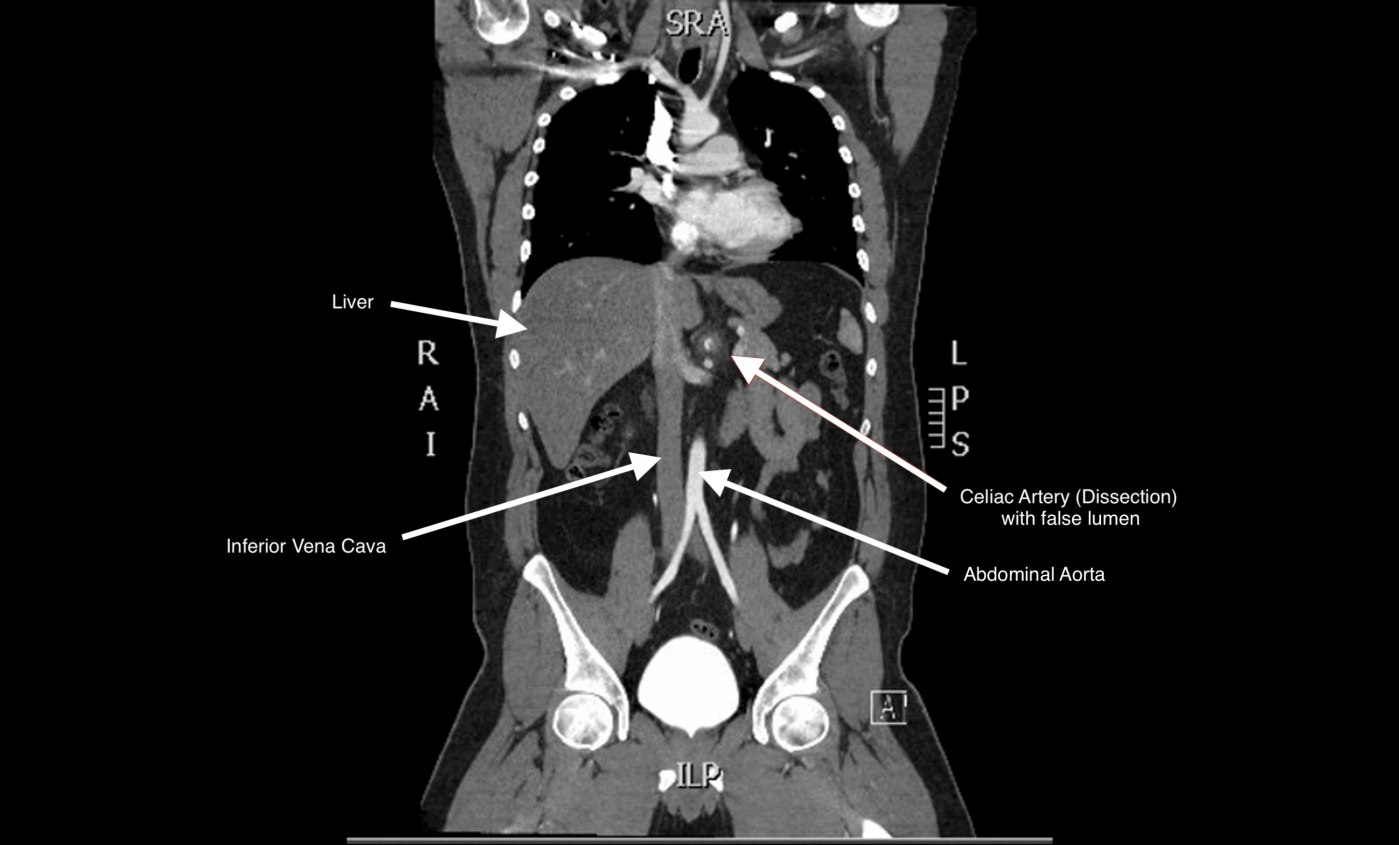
Computed tomography scan in coronal view
